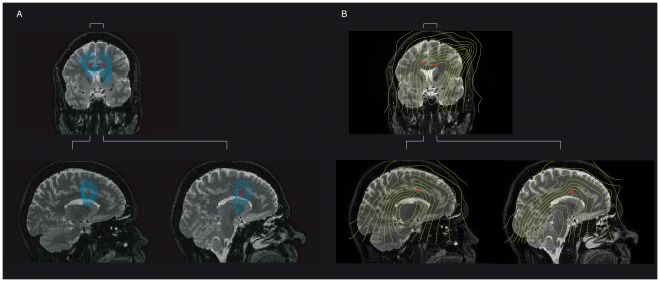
Comparison of the accuracy of using null and conventional beamformers for the localization of known locations of DBS electrodes. A) We used the null beamformer to localize the DBS electrodes when the stimulator was ON at 130 Hz. The coronal view of the lowest electrodes as localised on the patient’s MRI (red markers) compared with the overlay of contours of the estimated power using the null-beamformer. Two sagittal slices through lower left electrode and sagittal view of the lower right electrode. The fit is especially good on the left side. B) Similar localization using the conventional beamformer method shows a less good fit. In particular the method is unable to localize both electrodes.
